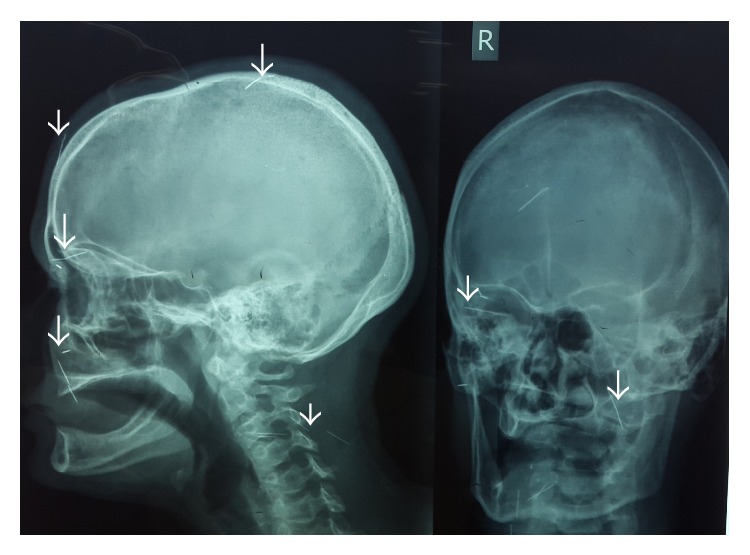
Skull X-ray showing at least eleven needles (some are arrowed).
